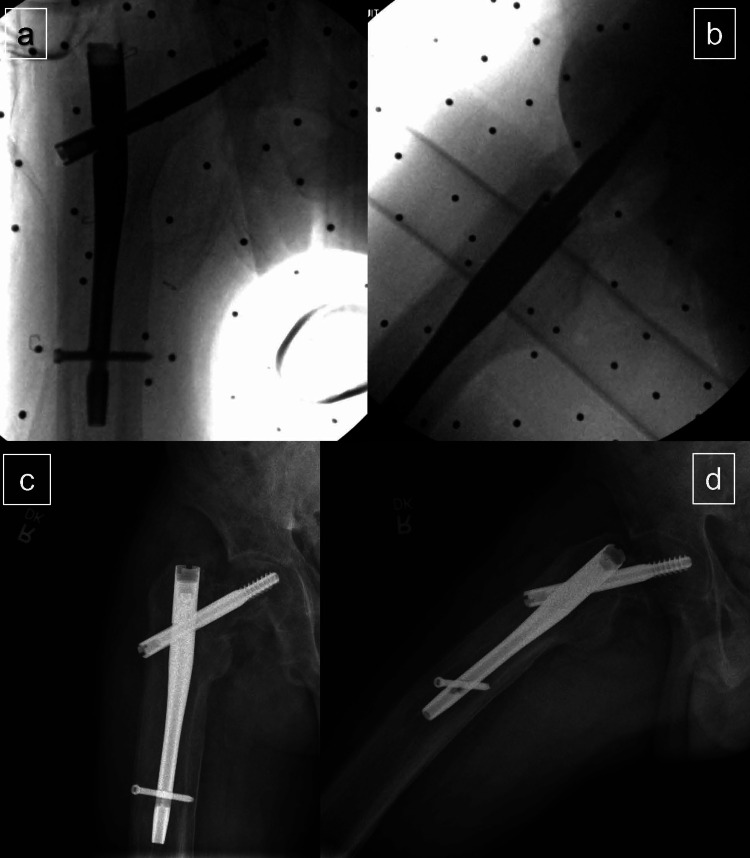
Post-operative fluoroscopy images after short cephalomedullary nailing anteroposterior (AP) (a) and cross-table lateral (b). One-year follow-up AP (c) and cross-table lateral (d) radiographs
